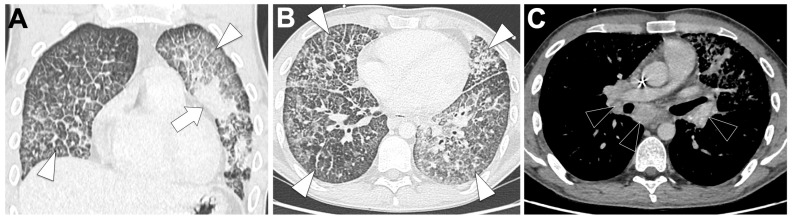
Representative baseline CT imaging findings in patients with RET-altered lung adenocarcinoma (LUAD). A 22-year-old man with a light smoking history presented with extensive bilateral lymphangitic carcinomatosis (white arrowheads) and a centrally located, spiculated solid mass in the left upper lobe (white arrow) on chest CT (A,B). On mediastinal kernel images, multiple enlarged bilateral mediastinal lymph nodes were also identified (black arrowheads) (C).
